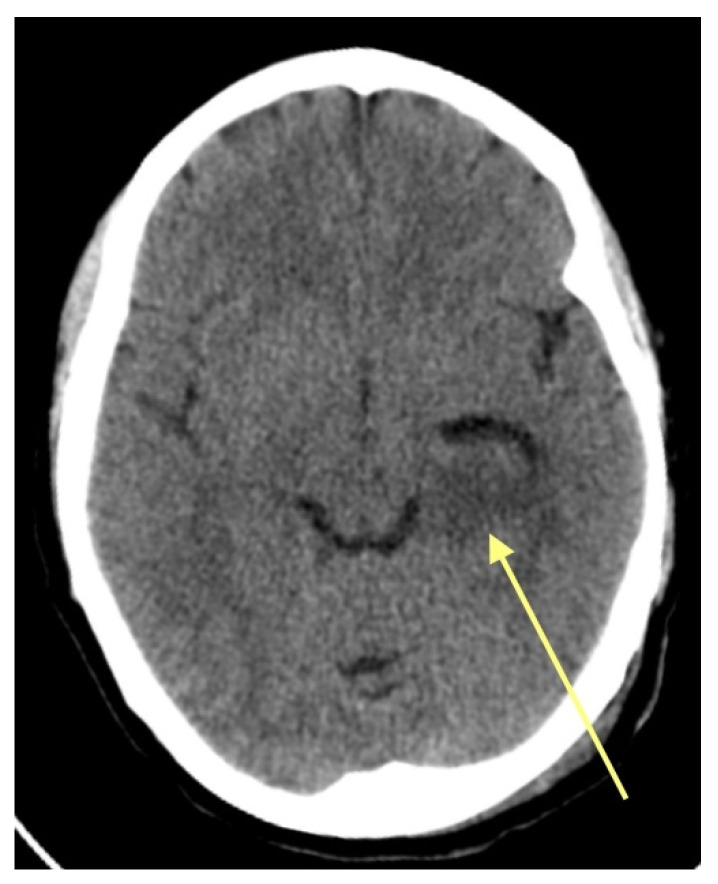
Axial CT image. The yellow arrow shows the hypodense area at the area of the left hippocampus.
